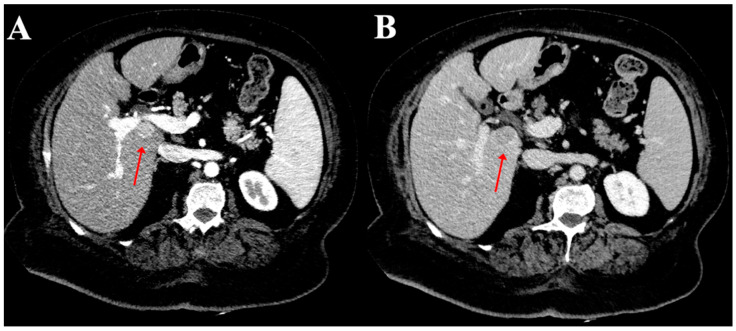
Computed tomography of the axial planes of an HCC nodule in the segment 1 (red arrows) showing hyperenhancement in the arterial phase (A) and wash-out in the portal phase (B).
